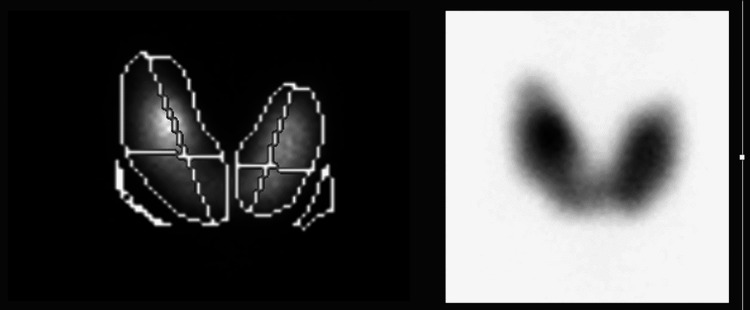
Thyroid scintigraphy with Tc-99m showing typical features of Grave’s disease and a diffusely enlarged thyroid gland with homogenously increased uptake and suppression of the background
